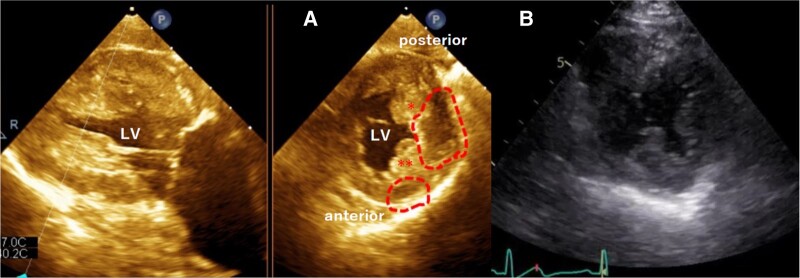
(*A*) Transoesophageal echocardiographic (TOE) trans gastric long-axis and short-axis images of the left ventricle shows hypoechoic areas in the anterolateral mid-wall of the left ventricle (circle) and in the posterior interventricular anterior () and posterior (*) papillary muscle (circle) that are different from normal myocardium. (*B*) The mass is indistinct on the short-axis image of the transthoracic echocardiogram (TTE).
